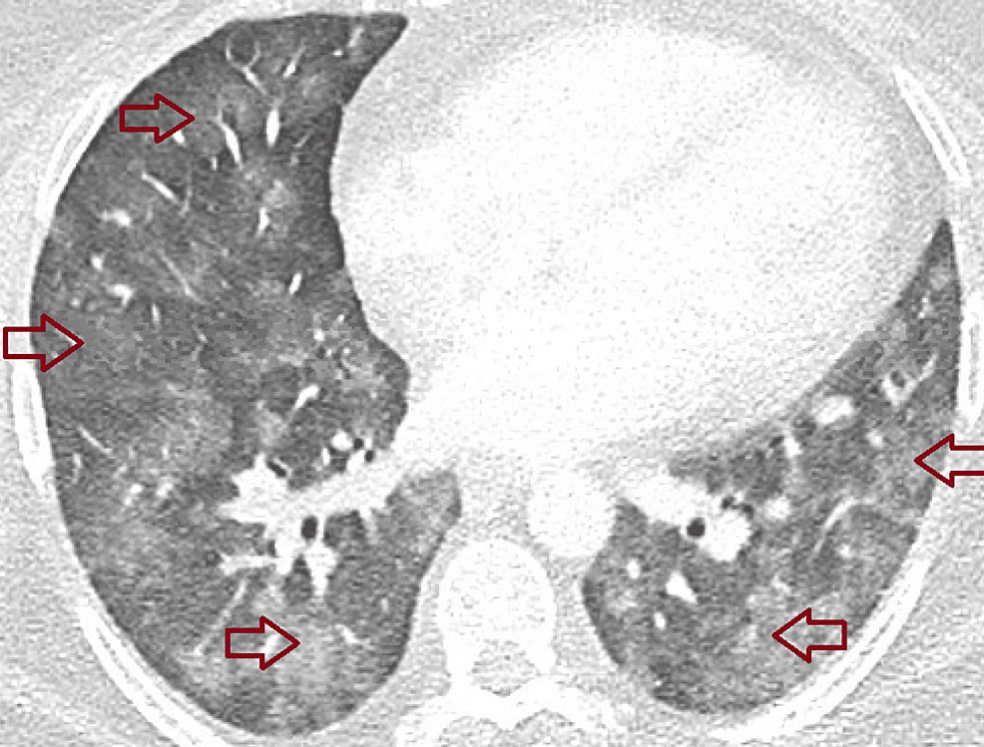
CT of the chest demonstrating multiple bilateral ground-glass opacities (red arrows).
